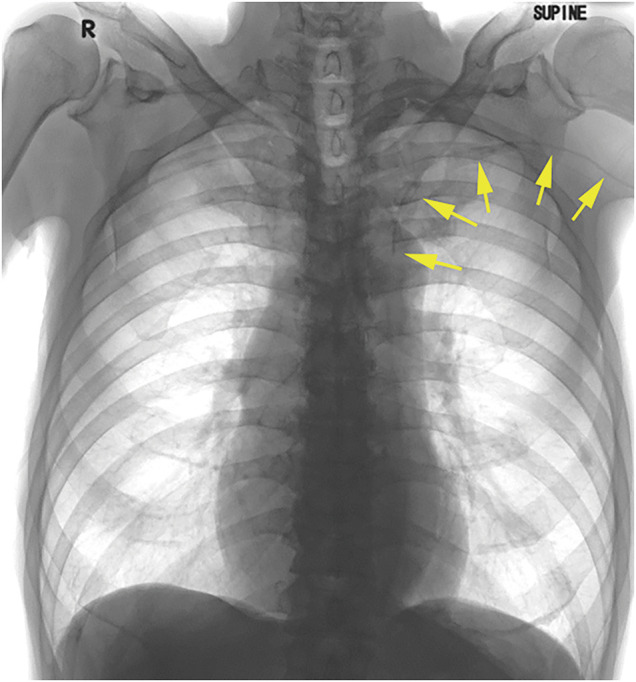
Chest radiograph after PICC port placement. The catheter tip was placed within the left superior vena cava through the PLSVC. Yellow arrows: catheter. PICC, peripherally inserted central catheter; PLSVC, persistent left superior vena cava
